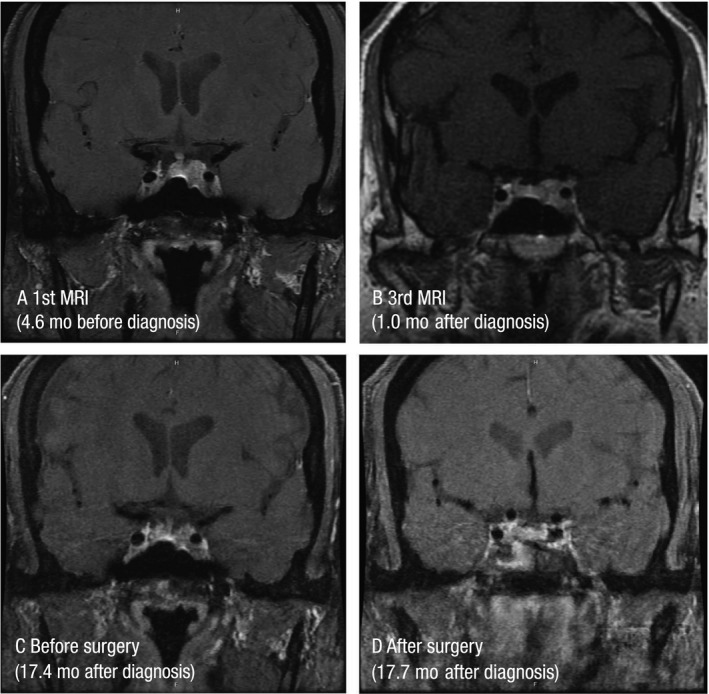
Magnetic resonance imaging of pituitary adenoma relative to the time of diagnosis and treatment of acromegaly: (A) 1st MRI, (B) 3rd MRI, (C) before surgery, and (D) after surgery. MRI, magnetic resonance imaging
